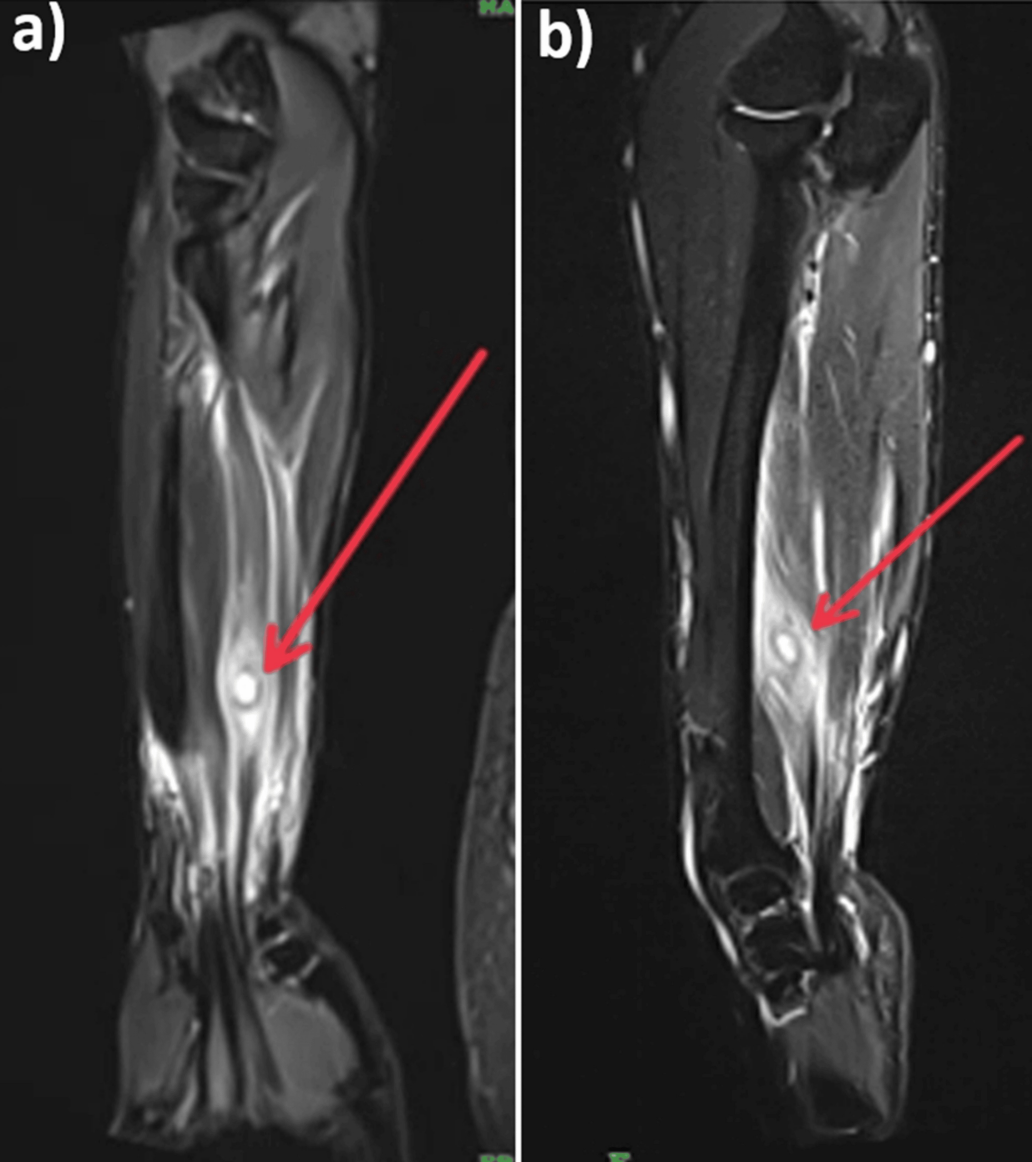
MRI of the right forearm. (a) Coronal cut. (b) Sagittal cut. MRI findings came out to be suggestive of a well-defined oval cystic lesion in the flexor digitorum profundus muscle showing scolex, partial calcification of the wall and a focal defect in the wall.
